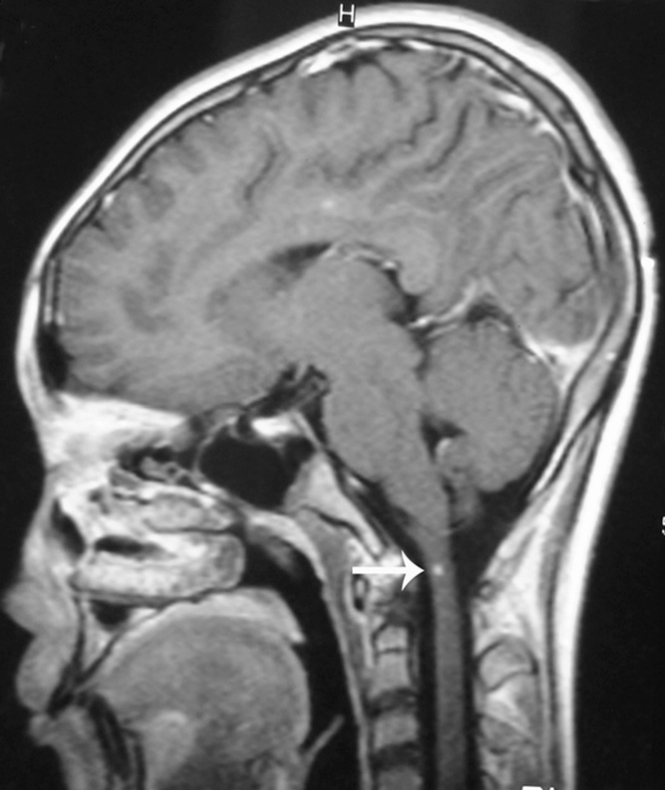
Spinal magnetic resonance imaging of the spine of the patient, showing a contrast-enhanced nodule in sagittal T1-weighted images after administration of gadolinilum.
